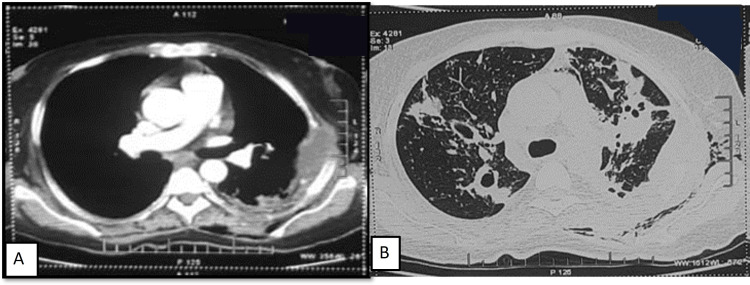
(A) Contrast-enhanced chest CT (mediastinal window) showing left lower lobe empyema with rib erosion and subcutaneous extension. (B) On lung window, bilateral upper lobe and right lower lobe infiltrates along with cavitation are seen.
